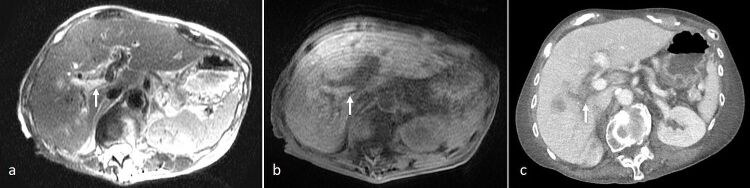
An 83-year-old lady with a history of rectal cancer, underwent radiofrequency ablation of a right hepatic lobe metastasis. She subsequently presented with fever and mild abdominal pain. NC-MRI was performed. (a) Axial *T*
